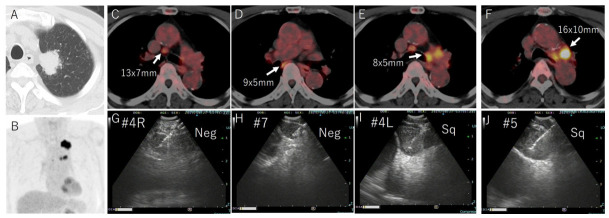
Combination of EBUS-TBNA and EUS-(B)-FNA revealed N2b disease. The primary tumor located in the left upper lobe was diagnosed as squamous cell carcinoma (A). PET-CT revealed abnormal FDG uptake in stations 4 L and 5, suggesting mediastinal nodal involvement (B–F). EBUS-TBNA was initially performed, which confirmed metastatic squamous cell carcinoma in station 4 L (G–I). Subsequently, the same bronchoscope was inserted through the esophagus, and EUS-(B)-FNA was performed for station 5, which revealed metastatic disease (J). Based on the involvement of multiple mediastinal nodal stations, the patient was diagnosed with N2b and subsequently underwent perioperative chemoimmunotherapy. Neg: negative; Sq: squamous cell carcinoma.
